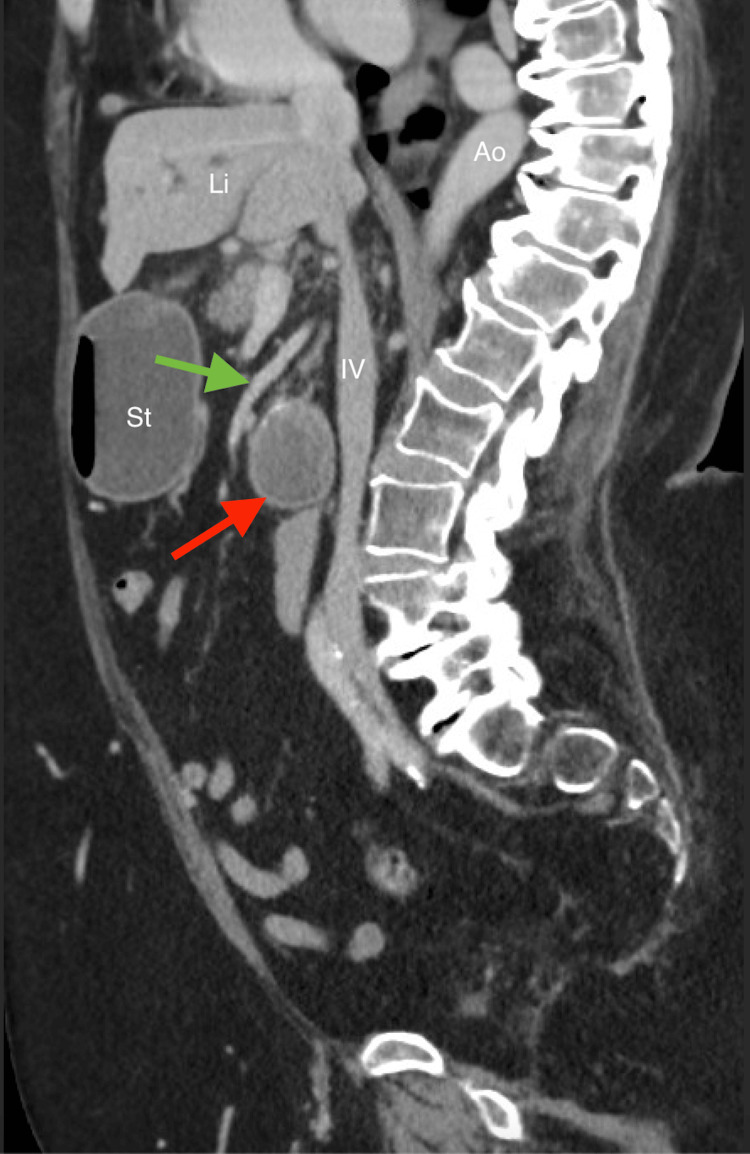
Contrast-enhanced sagittal CT of the abdomen demonstrates a large, rounded hyperdense calculus within the duodenal lumen (red arrow), consistent with an ectopic gallstone, resulting in adjacent stomach (St) is distention. The superior mesenteric artery (SMA; green arrow) is seen coursing anterior to the duodenum, with the aorta (Ao), the inferior vena cava (IV) and liver (Li) labeled for anatomic orientation.
